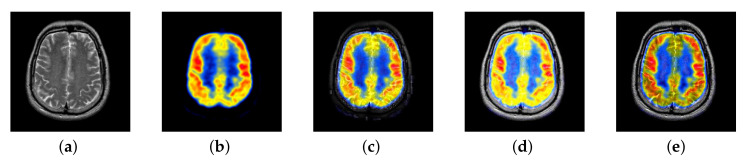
Source images and fused medical images obtained by different algorithms: (a) MRI, (b) positron emission tomography (PET), (c) DenseNet, (d) traditional intuitive fuzzy processing (IFP), and (e) FusionNet.
